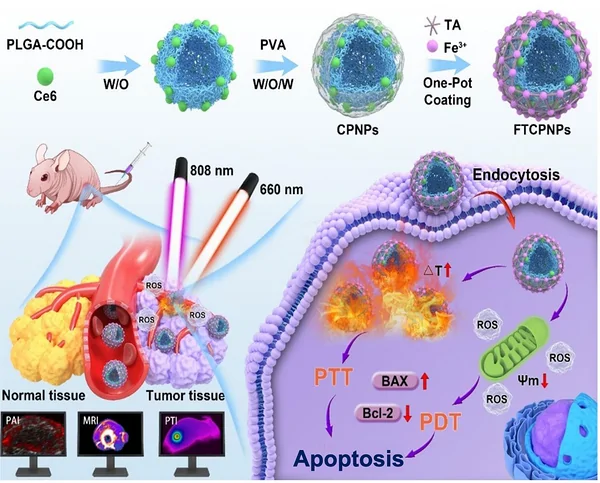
FTCPNPs synthesis and combination effects of PTT/PDT against UM tumors. Following tumor accumulation, FTCPNPs enable MRI and PAI imaging and, under 808 nm and 660 nm irradiation, induce heat and ROS generation, respectively. The combined treatment promotes mitochondrial dysfunction, Bax upregulation, Bcl‐2 downregulation, and apoptosis, resulting in greater antitumor efficacy than either PTT or PDT alone. Reproduced with permission [211]. Copyright 2023, Springer.
